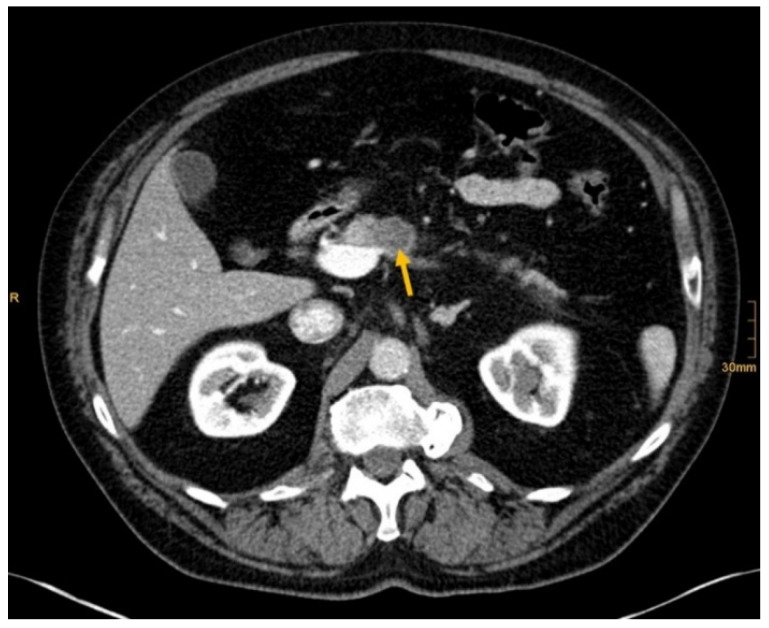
Pancreatic cancer (arrow). Correctly identified by one radiologist without and by all three radiologists with given clinical information (Conspicuous pancreas lesion seen in abdominal ultrasound. Laryngeal carcinoma several years ago.).
